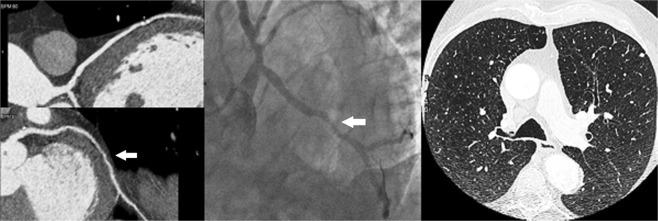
A case of coronary artery disease detection. Cardiac CT revealed a significant 80% stenosis (*white arrow*) of the left circumflex artery (left panel), which was confirmed (*white arrow*) at invasive coronary angiography (middle panel). CT images of the lungs did not show any pulmonary nodule (right panel).
